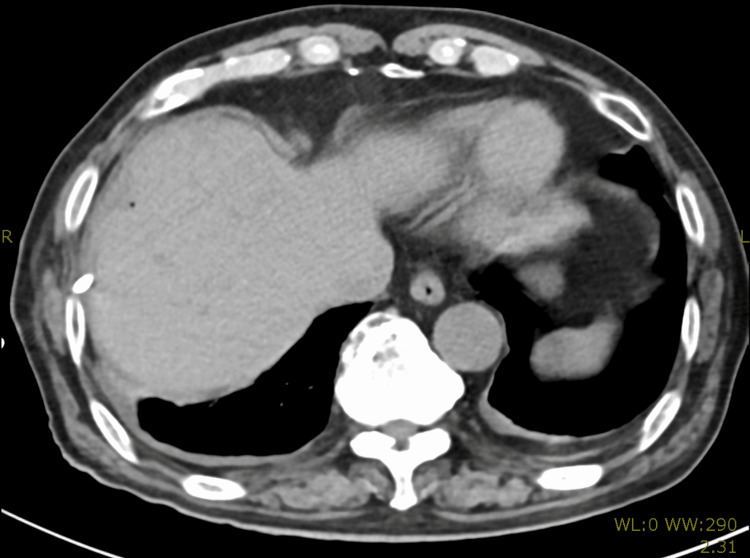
Computed tomography on hospital day 39 showing complete resolution of the subcapsular abscess.
